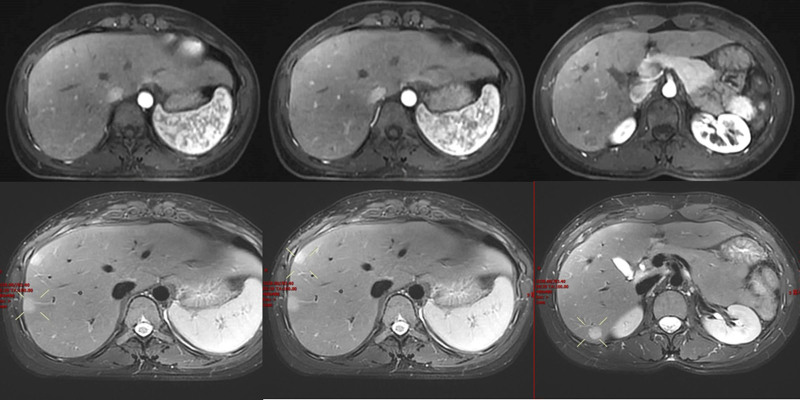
Upper abdominal magnetic resonance imaging in September 28, 2023: T1-weighted image arterial enhancement stage and T2-weighted image nonarterial enhancement stage.
